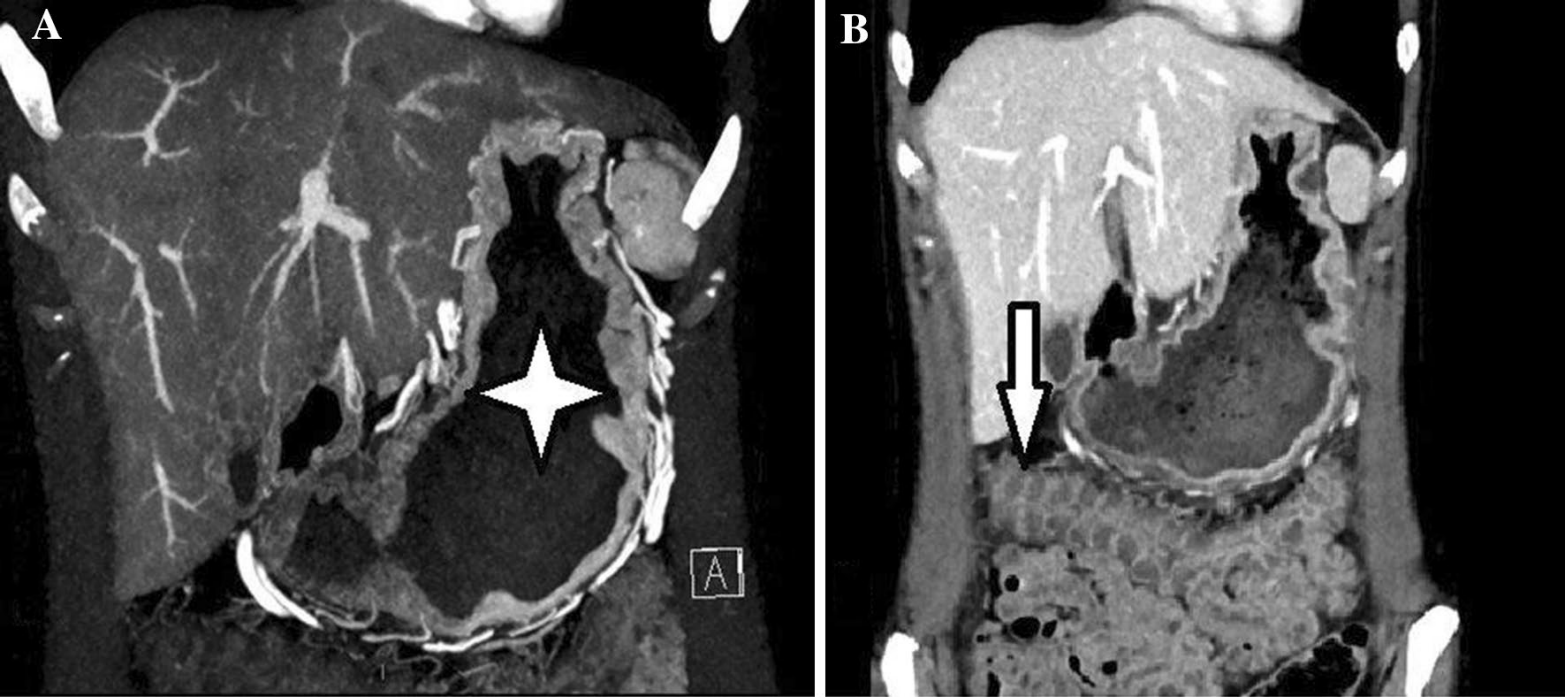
CT angiographic phase with marked hyperemic rugal folds in the body of the stomach (highlighted by star; a). Thickened and oedematous colonic haustra project into the lumen of transverse colon in portal venous phase, caused by hypoalbuminemia (highlighted by arrow; b)
